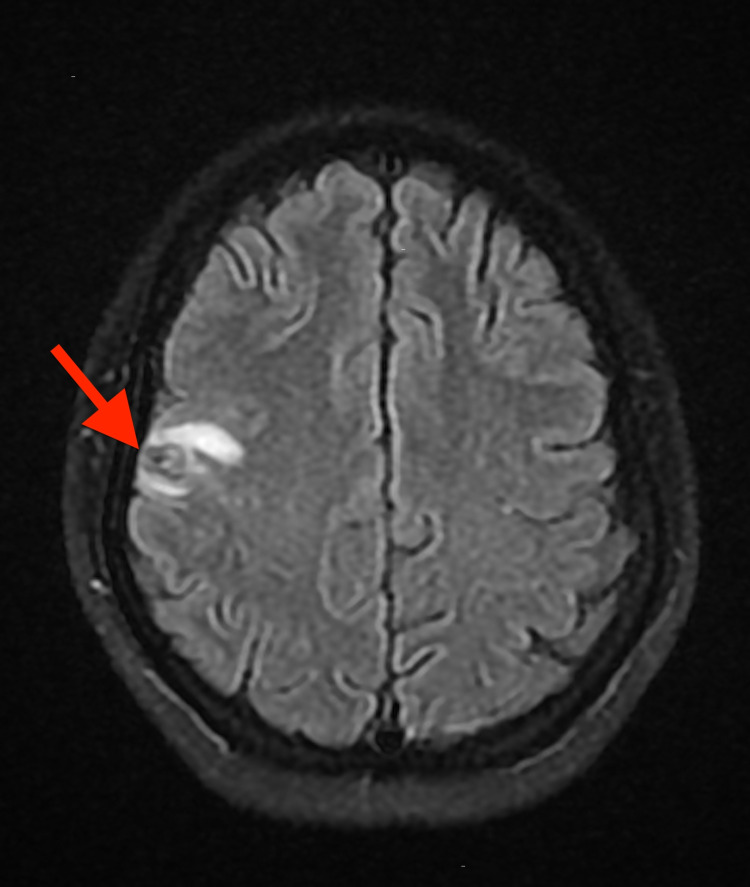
Axial brain MRI showing decrease in size of cystic mass (red arrow).
